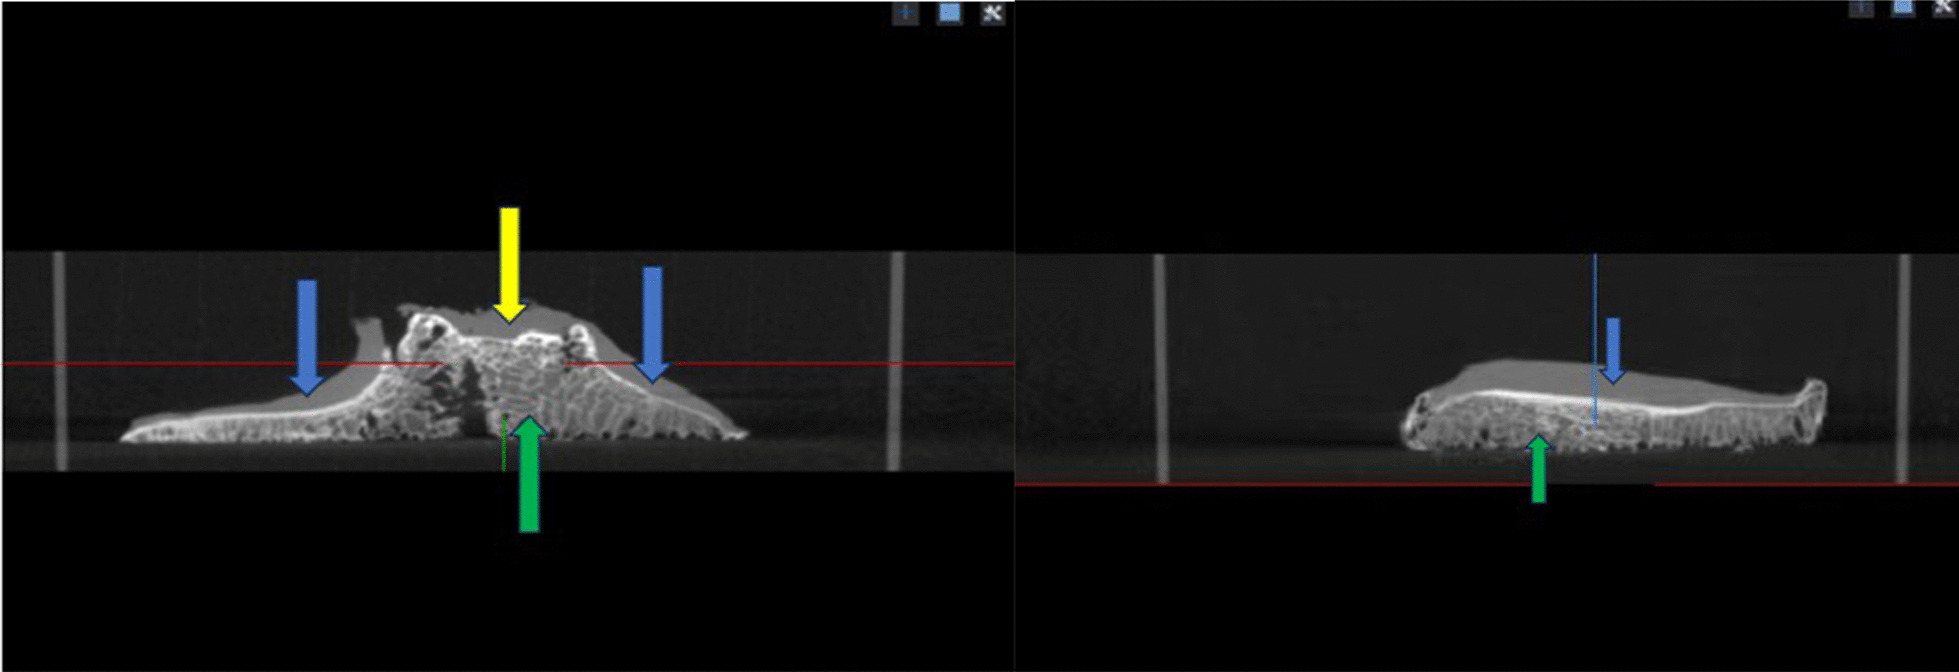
CBCT of the natural tibial plateau cartilage. The picture on the left is the coronal view, and the picture on the right is the sagittal view. Green: bone; blue: cartilage; yellow: residual ligament stumps (soft tissue)
